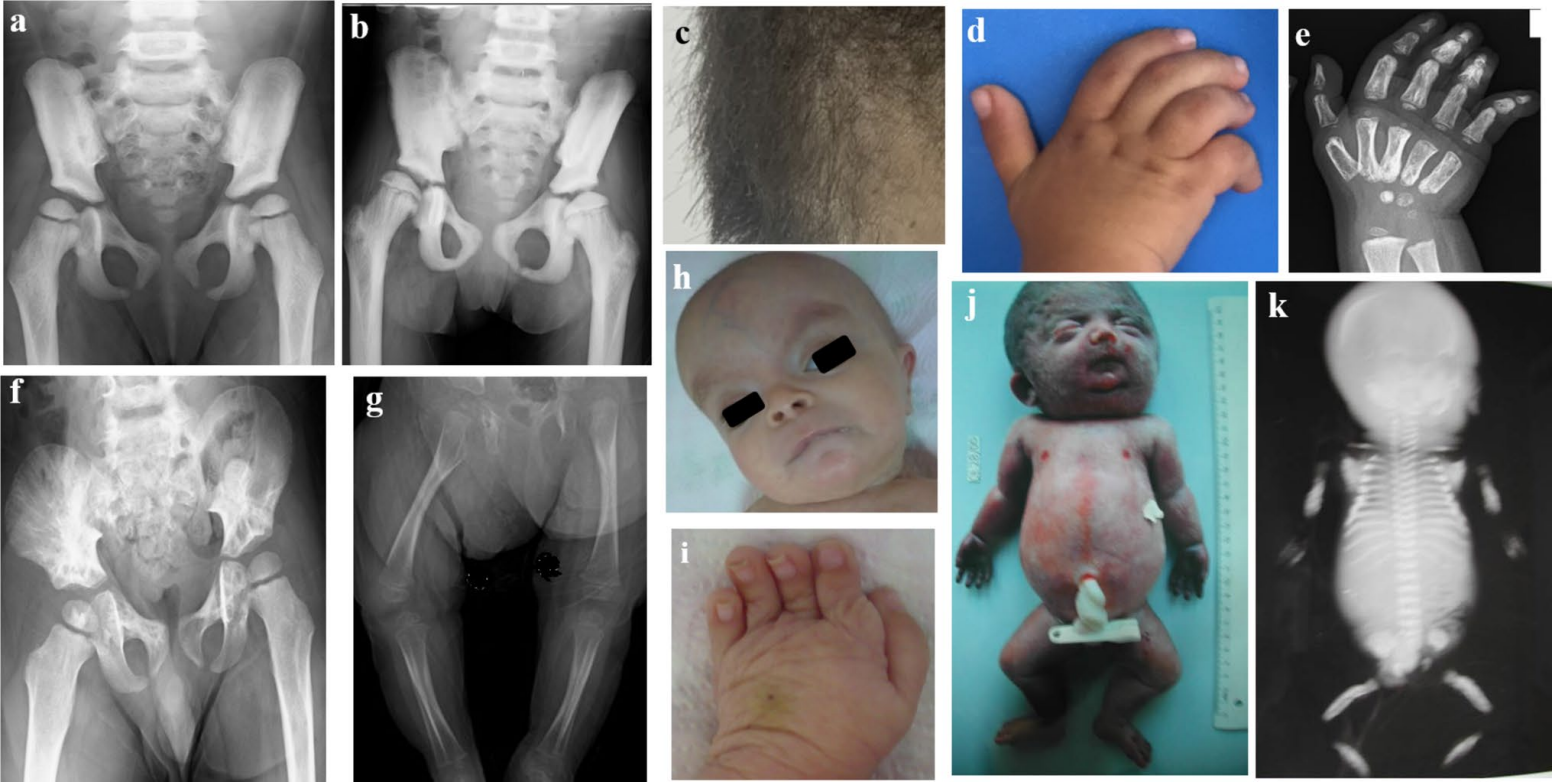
Patients with ultra-rare osteosclerotic disorders. P31 with Trichothiodystrophy-1 (a, c) at 3.8 years of age and 9.5 years of age (b). Note sparse, brittle hair, and diaphyseal and pelvic sclerosis, irregular acetabulum. P32 at 34 months of age with melorheostosis (d–f), right elbow contracture with dripping wax-like lesions and hyperostosis on pelvis radiograph. P33 with Lenz-Majewski hyperostotic dwarfism (g–i) at 6 months of age with prominent forehead, hypertelorism, severe brachydactyly with partial syndactyly, diaphyseal sclerosis, and metaphyseal osteopenia. A fetus with perinatal Caffey disease (j, k) showing generalized osteosclerosis and loss of cortex medulla border
